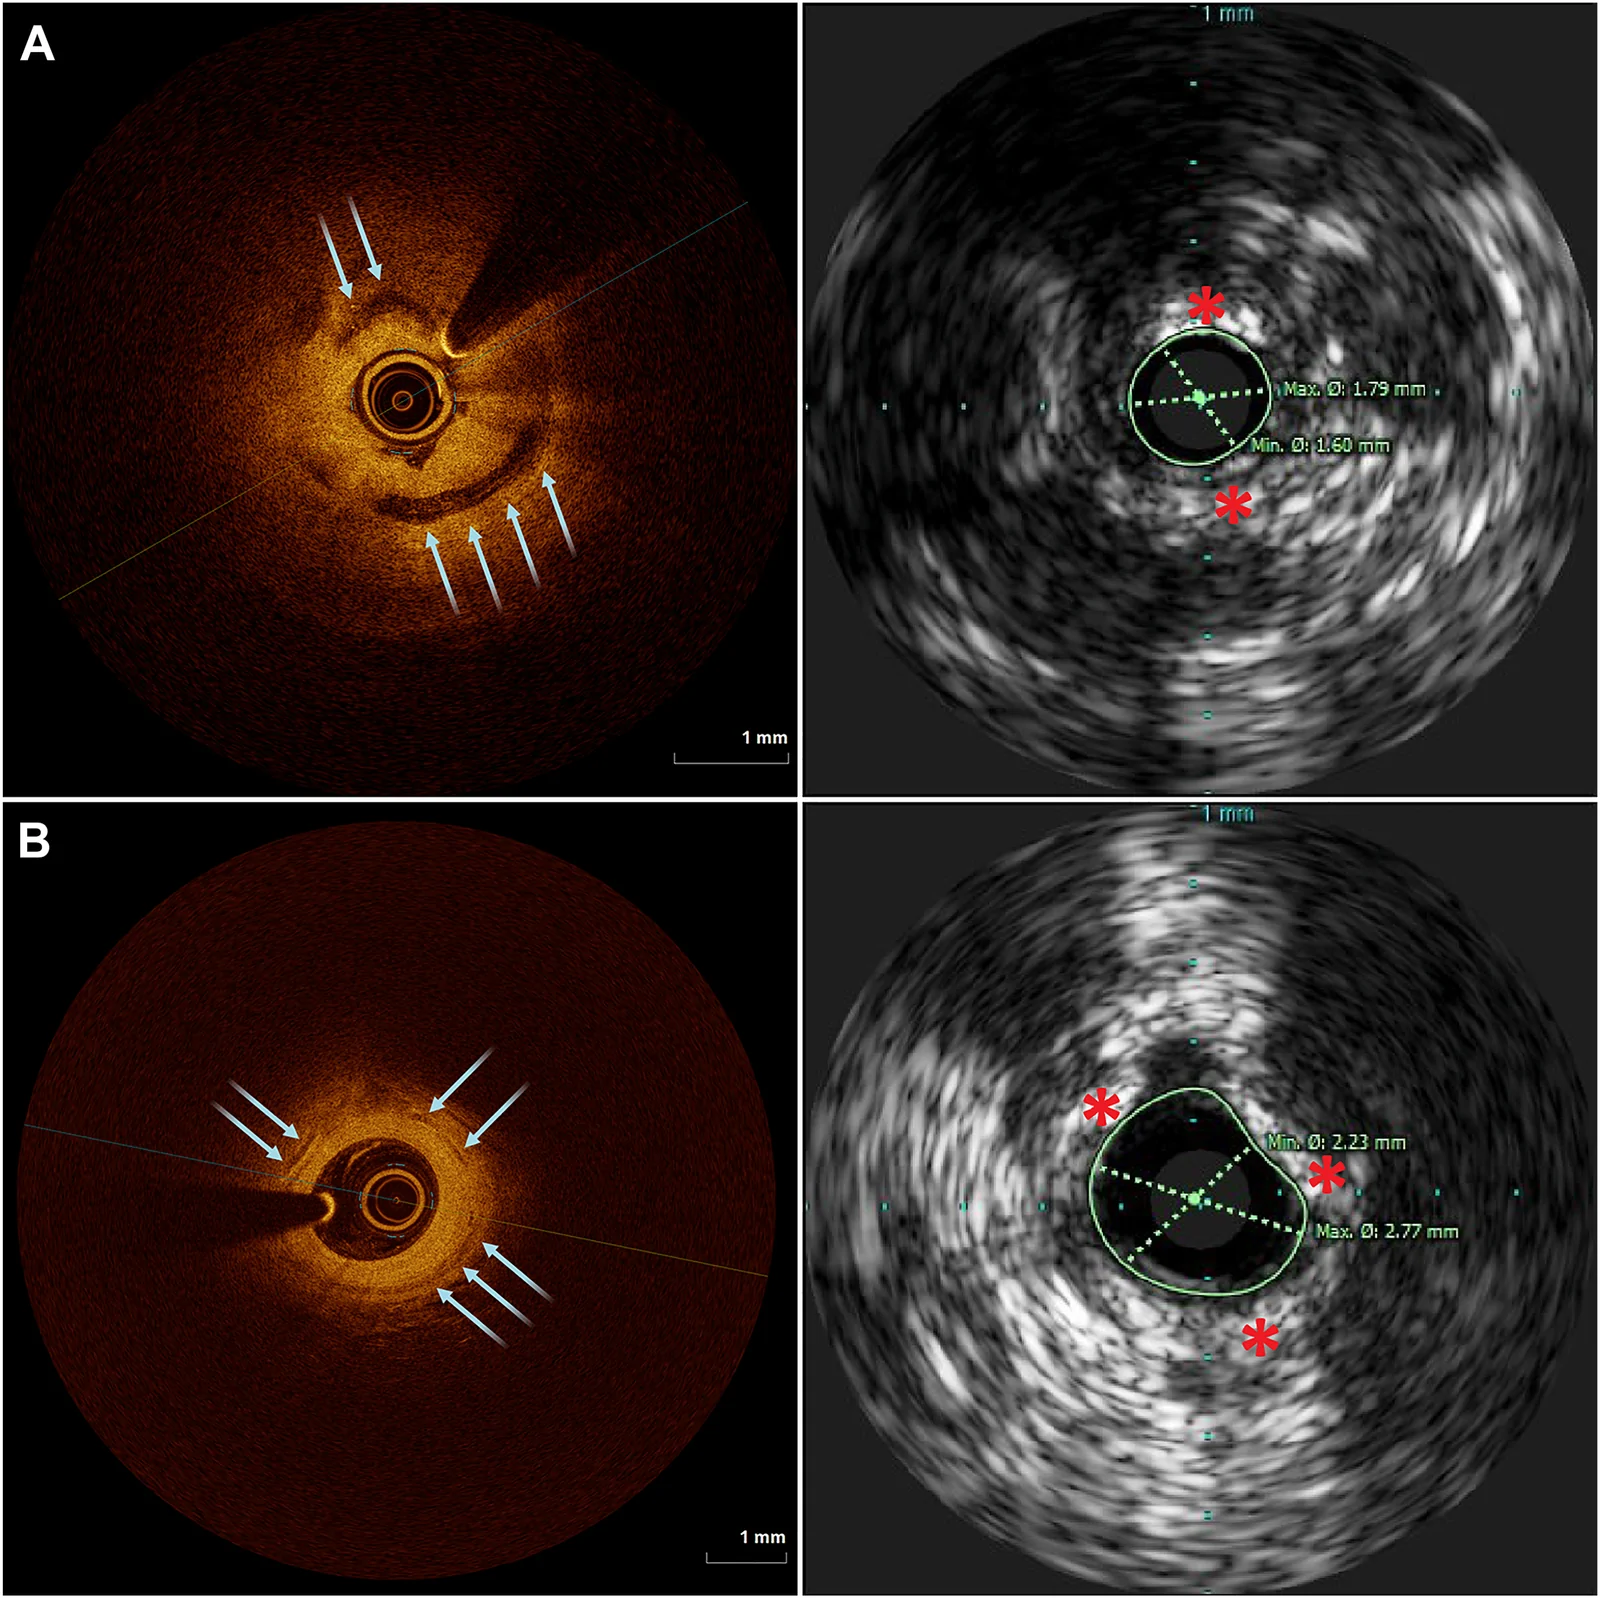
Examples oftwo different subjectswithcorresponding optical coherence tomography and intravascular ultrasound MLF with calcium. Blue arrows denote calcium noted on optical coherence tomography, and red asterisks mark calcium on intravascular ultrasound.
